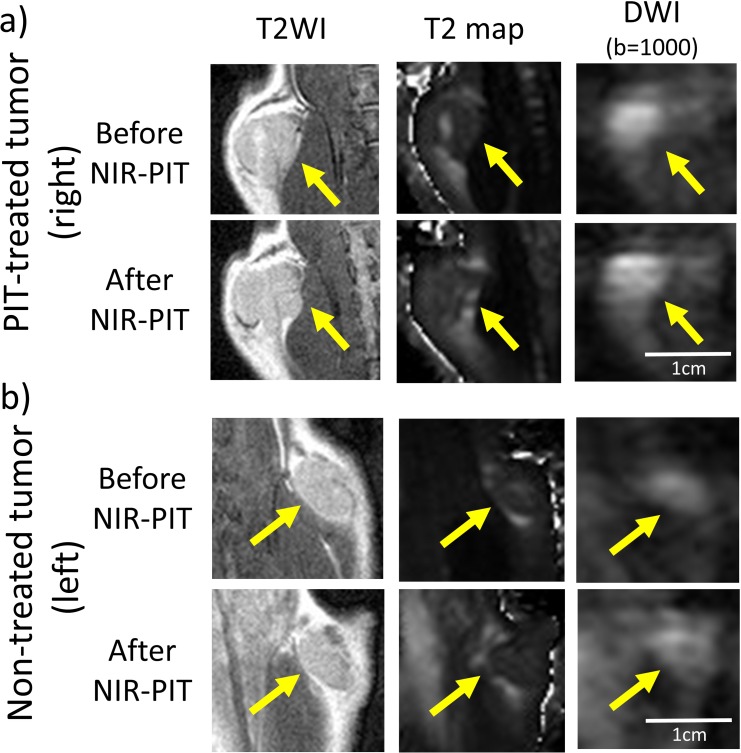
T2WI, T2 map, and DWI with b-value of 1000 sec/mm^2^ of a NIR-PIT-treated a. or a non-treated control tumor b. before and after NIR-PIT. Arrow indicates the tumor.
